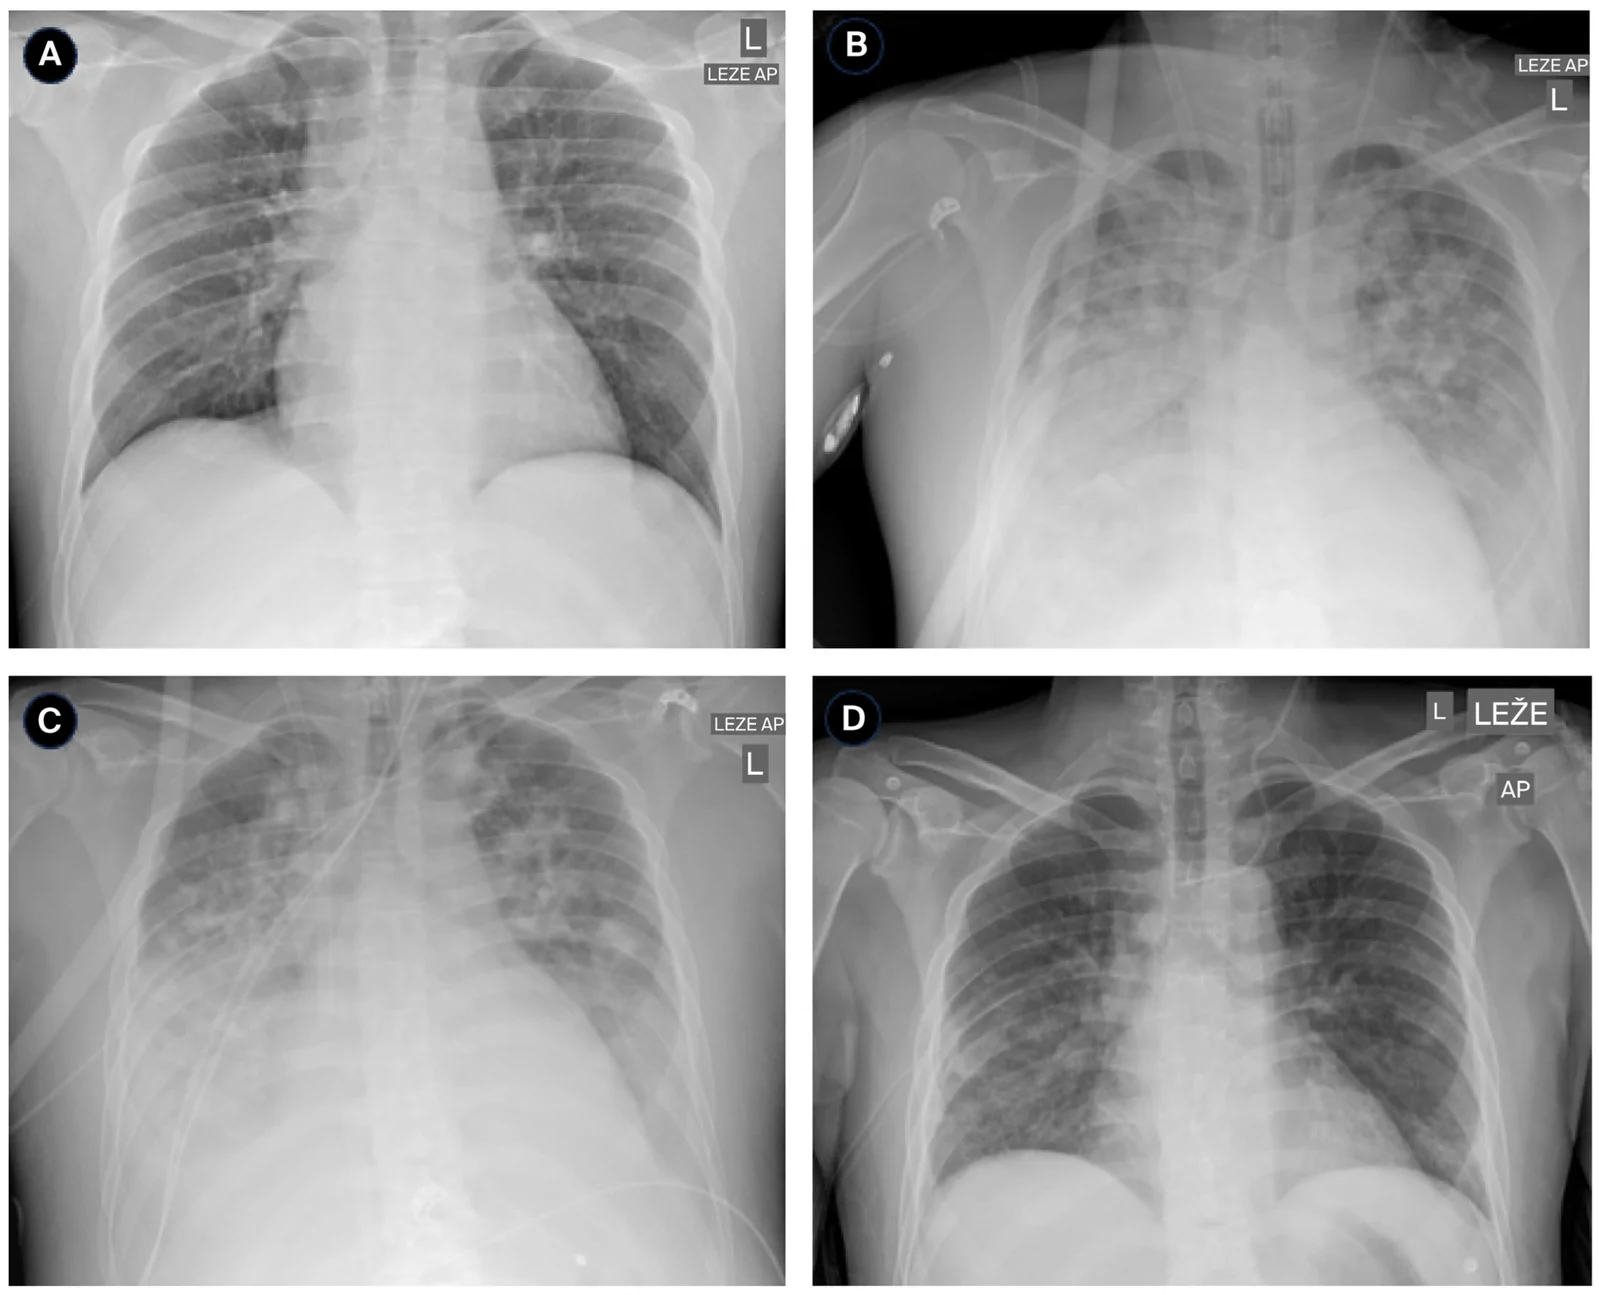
Serial chest radiographs. (A) Pre-relaparotomy radiograph obtained before aspiration, showing no acute pulmonary abnormality. (B) Intraoperative radiograph obtained after VV-ECMO initiation, showing diffuse bilateral pulmonary opacities and initial cannula malposition. (C) ICU radiograph obtained after cannula repositioning, showing persistent diffuse bilateral pulmonary opacities. (D) Post-decannulation radiograph showing marked interval improvement in bilateral pulmonary opacities. “LEŽE AP” denotes a supine anteroposterior radiograph; “L” indicates the patient’s left side.
